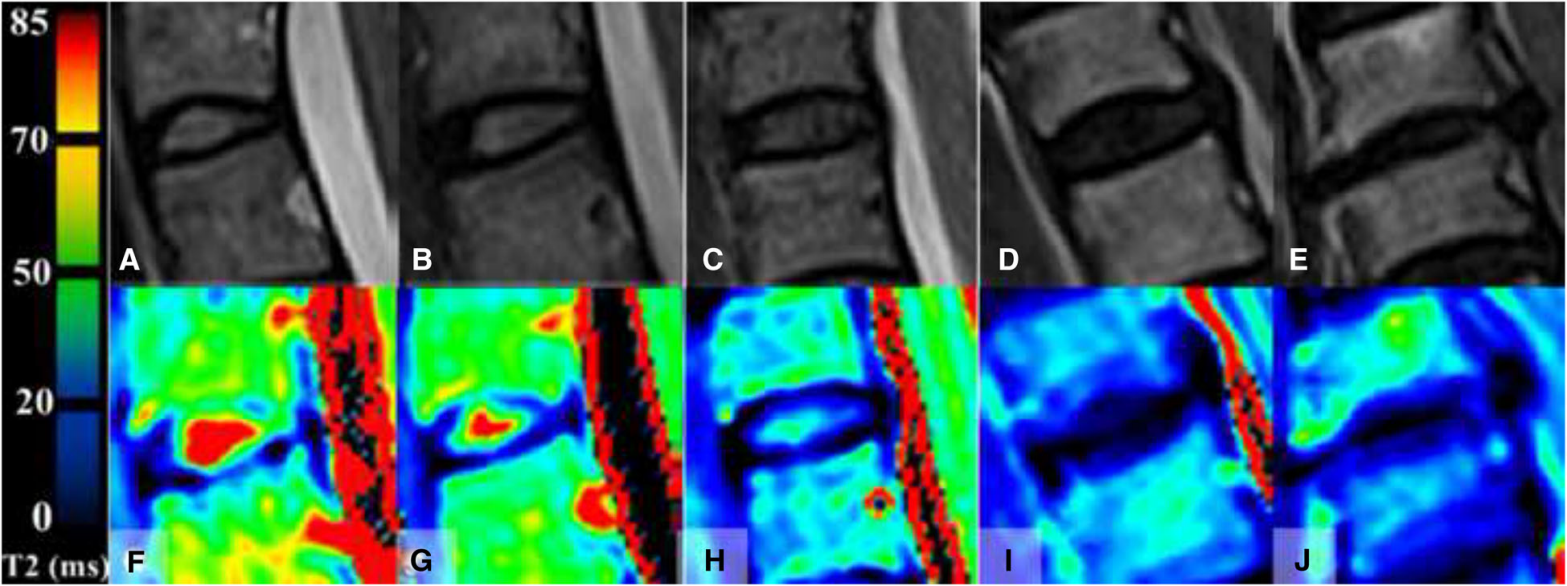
Sagittal T2-weighted FSE images and corresponding T2 maps of discs with different Pfirrmann grades. a and f grade I; (b and g) grade II; P (c and h) grade III; (d and i) grade IV; (e and j) grade V
